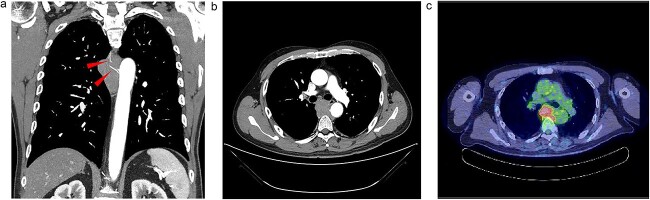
Radiologic findings. (a) A 15-cm soft tissue shadow in the posterior mediastinum of Th6-10, presenting as a multinodular mass. (b) Thoracic paravertebral soft tissue mass in the axial view. (c) Image of positron emission tomography-CT.
